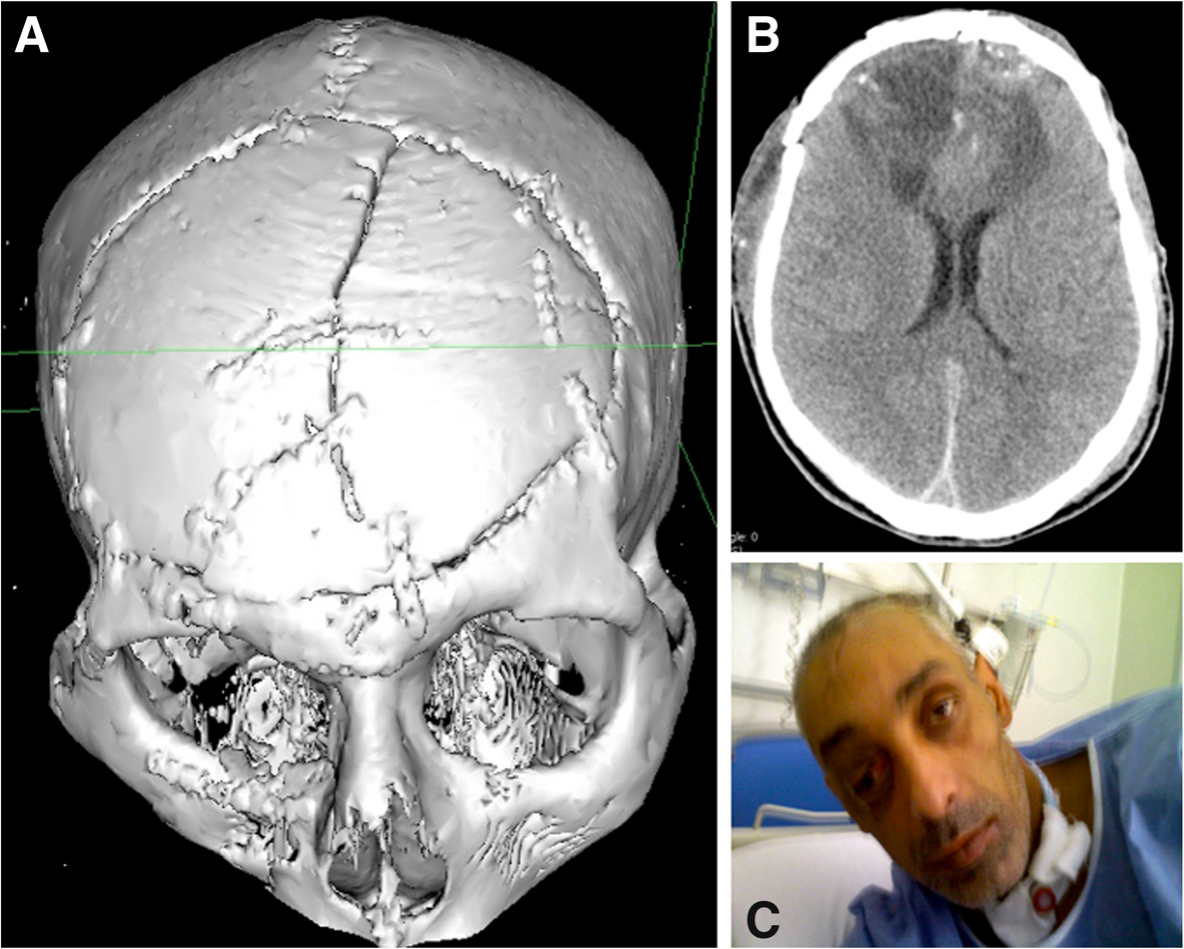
Clinical and radiological outcome. A: 3D CT scan view showing the final reconstruction of the fractured frontal bone. B: CT scan performed one month after injury, demonstrating the hypodensity of both frontal lobes as chronic consequences of penetrating injury. C: Patient at discharge with paresis of right superior rectus muscle and ptosis.
